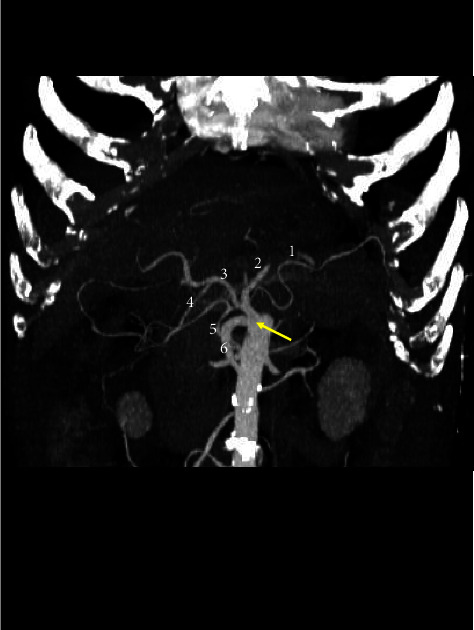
Three-dimensional volume rendering CT image showing a complete CMT (arrow). The celiac branches visible include the splenic (1), left gastric (2), common hepatic (3), and gastroduodenal arteries (4). The mesenteric branches visible include the inferior pancreaticoduodenal (4) and superior mesenteric ramifications (5).
